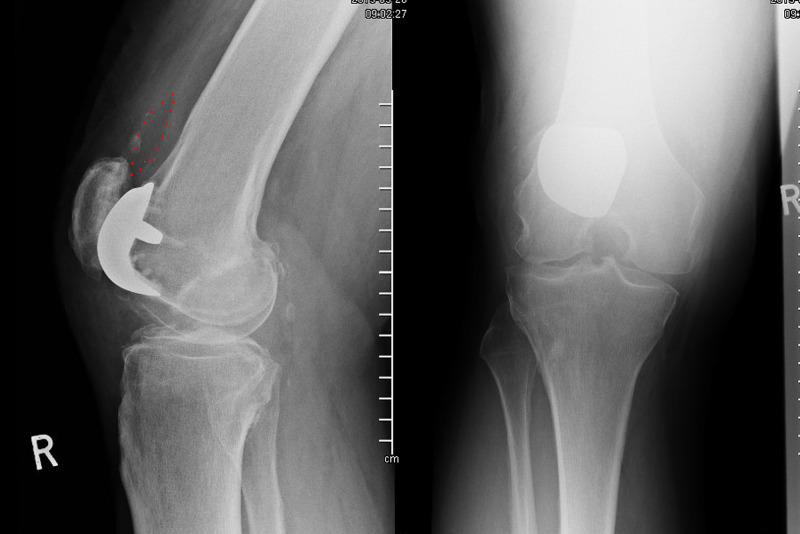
Pre-operative radiographs of the right knee displaying loose patella button (outlined on lateral view). Anteroposterior (right) and lateral (left) non-weight bearing radiographs of the right knee. The patella is lying directly on the femoral component of the implant and outlined in red is the shadow of the dislocated patella button of the failed FPV arthroplasty, lying within the suprapatellar pouch.
